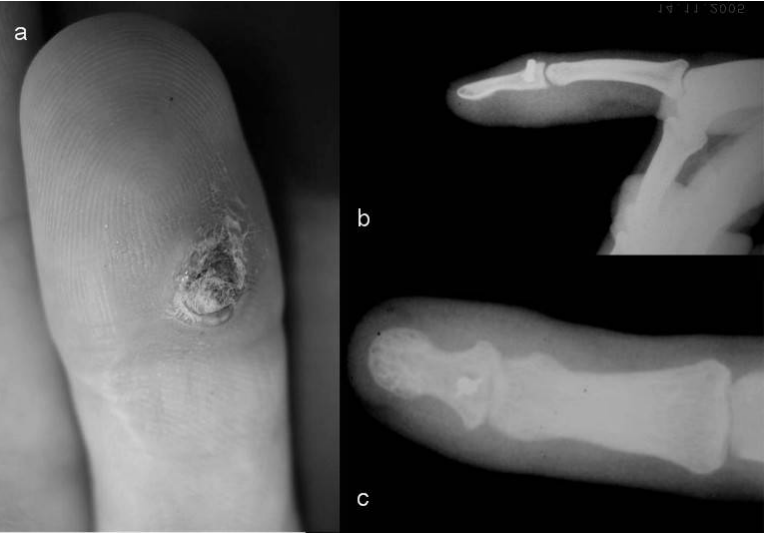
(a) recurrent ulcer on the palmar side of distal phalange of index finger, (b) lateral and (c) anterioposterior x-rays.
